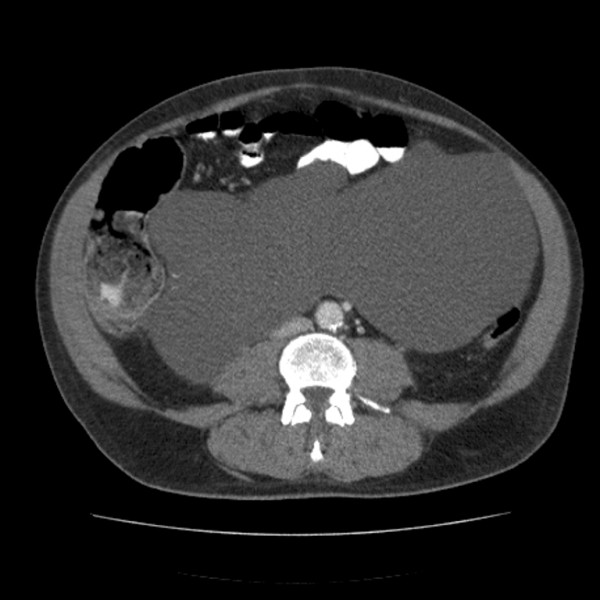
CT scan performed on initial admission showing large intra-abdominal cystic lesion.
